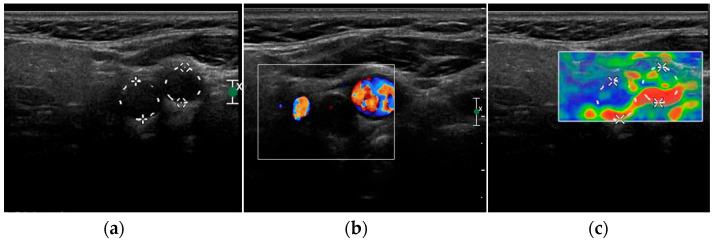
Grey-scale (a), Color Doppler (b) and elastography (c) aspect of a carotid body paraganglioma.
